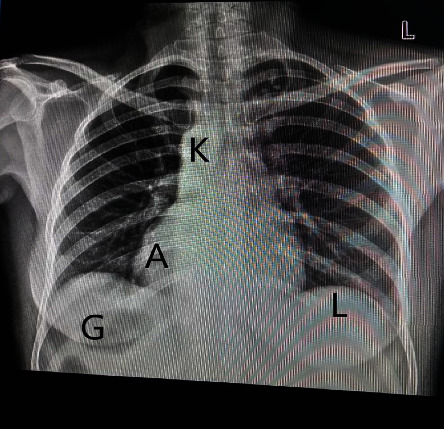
A posteroanterior chest X-ray reveals the cardiac apex (A) and aortic knuckle (K) pointing to the right, the gastric bubble (G) in the right upper quadrant, and the liver (L) shadow in the left.
